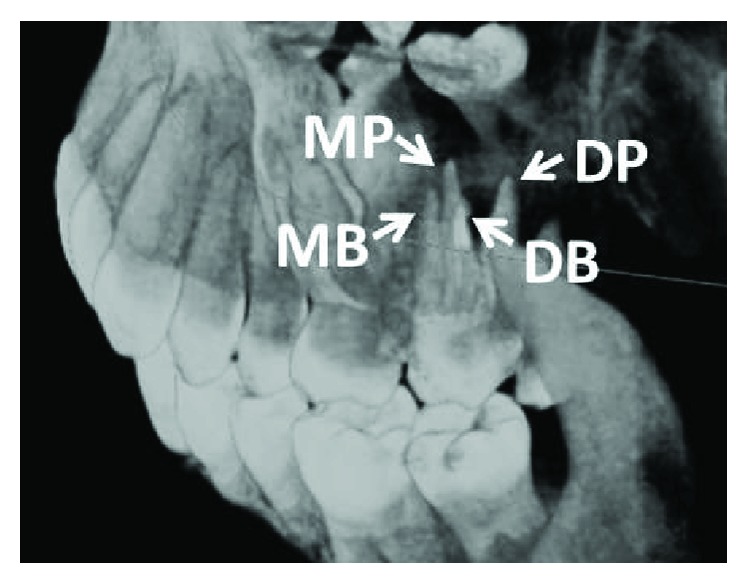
Cone beam computed tomographic 3D reconstruction of the treated tooth from a disto-buccal-apico angulation verifies the rare anatomy of four roots. MB: mesiobuccal; DB: distobuccal; MP: mesiopalatal; DP: distopalatal.
